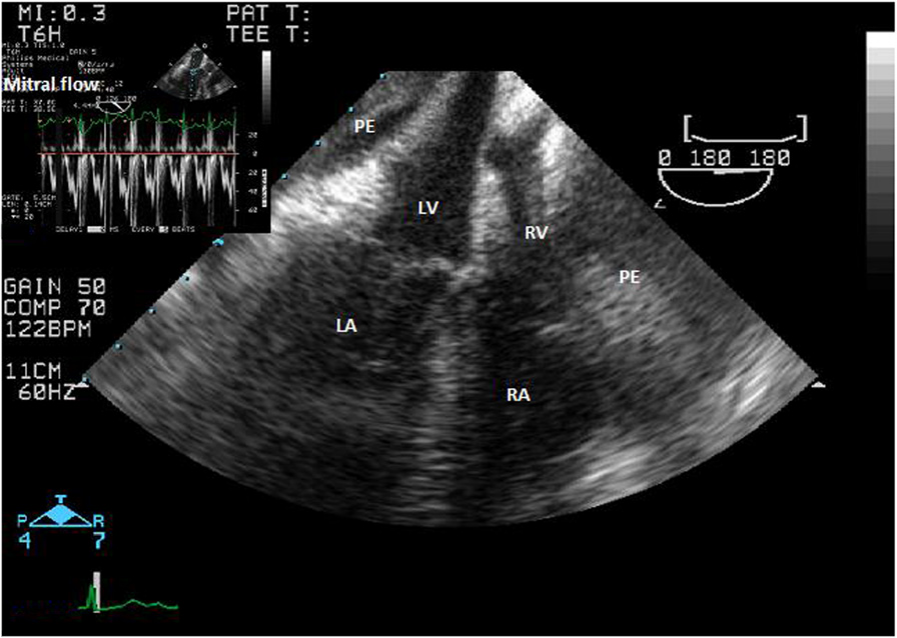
Transesophageal echocardiography. Cardiac tamponade. Pericardial effusion with collapse of left and right ventricles and significant respiratory variations of the transmitral flow velocities (left upper corner) – typical for cardiac tamponade. PE – pericardial effusion, LV - left ventricle, RV – right ventricle, LA - left atrium, RA – right atrium.
